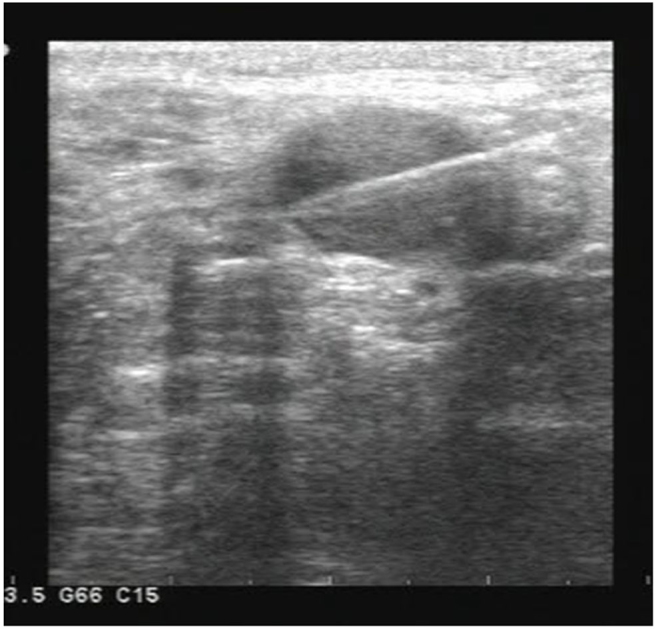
Ultrasound-guided biopsy findings of a left popliteal lymph node in the patient shown in Fig. 3.
